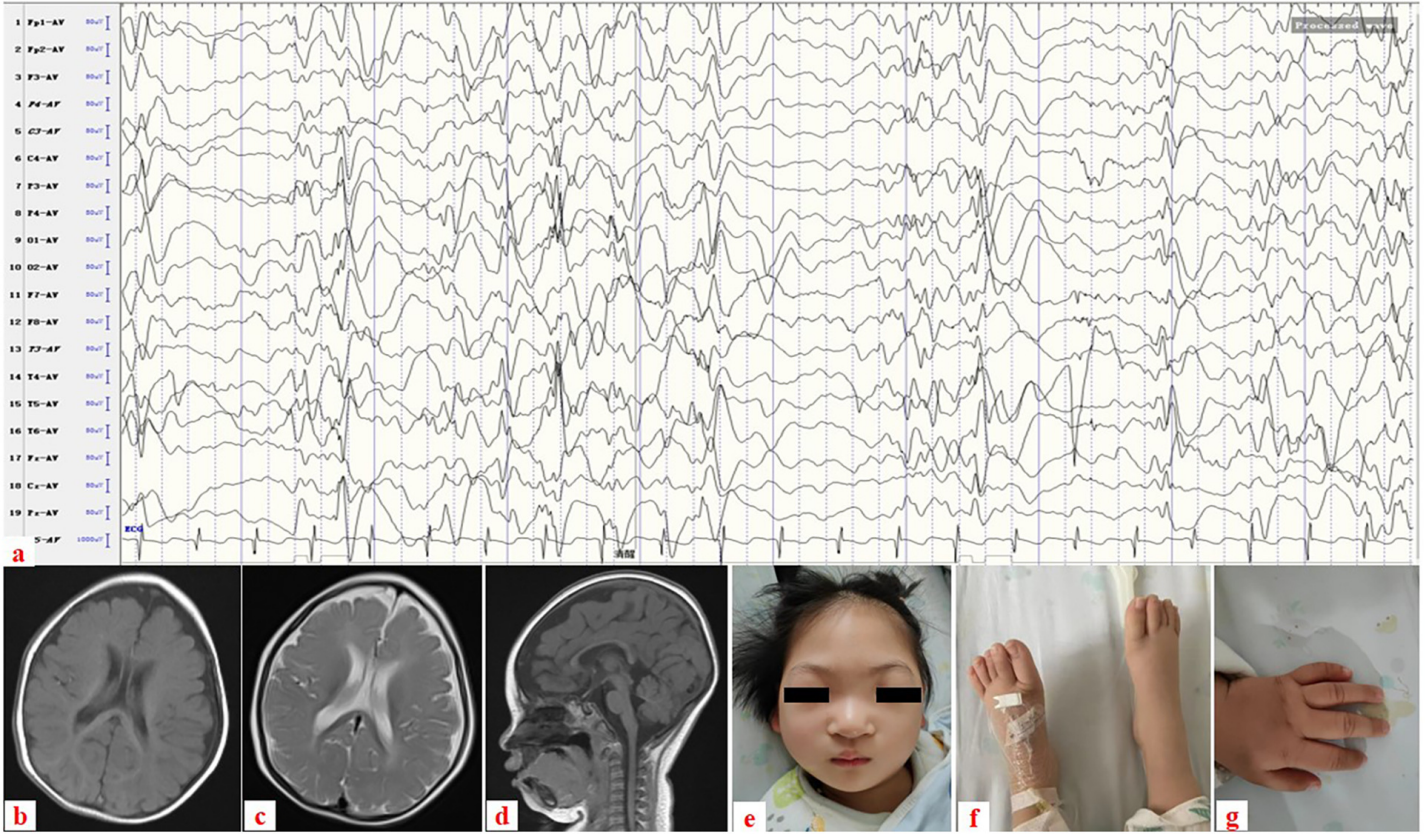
(a) Electroencephalogram showing hypsarrhythmia. (b–d) Brain MRI taken at 7 months’ old: (b) axial image in T1-weighted image, (c) axial image in T2-weighted image, and (d) sagittal image in T1-weighted image reveal cerebral atrophy with subsequent enlargement of the subarachnoid spaces. (e–g) Clinical photograph taken at 1 year and 6 months’ old: (e) Dysmorphic facial features with microcephaly and ocular hypertelorism, (f) normal feet, and (g) normal left hand.
